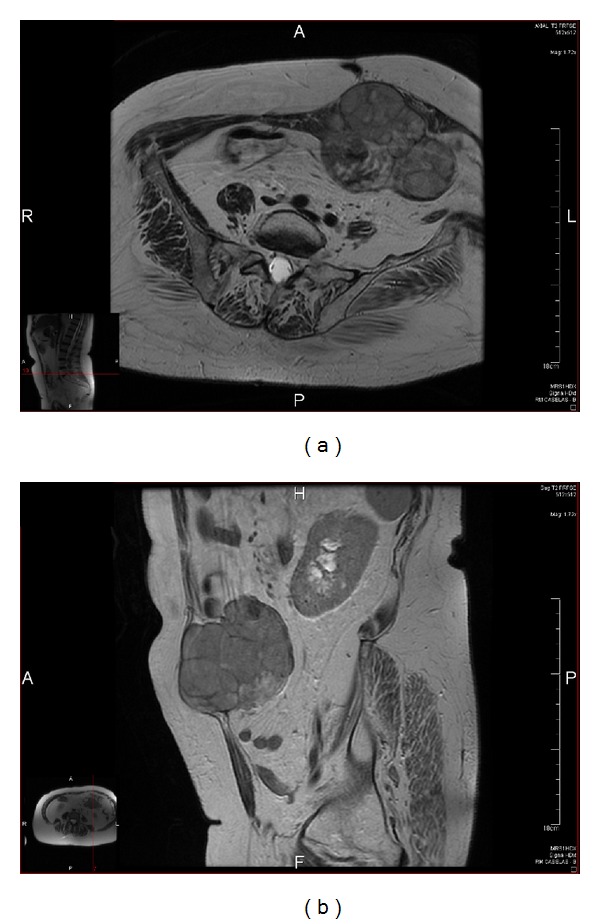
MRI demonstrates the tumour on the left side of the anterior abdominal wall protruding into the abdominal cavity. (a) Axial T2-weighted image and (b) sagittal T2-weighted image.
